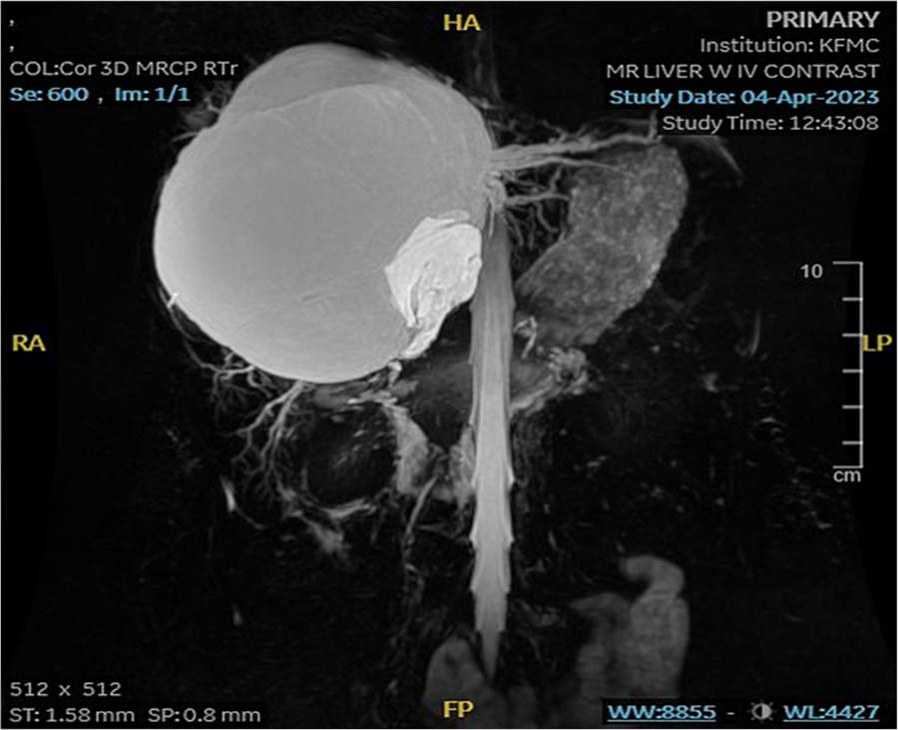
MRI of the liver: Demonstrating smaller bright T2 signal intensity lesions without post-contrast enhancement were observed in segment 7 and segment 6, consistent with cysts.
